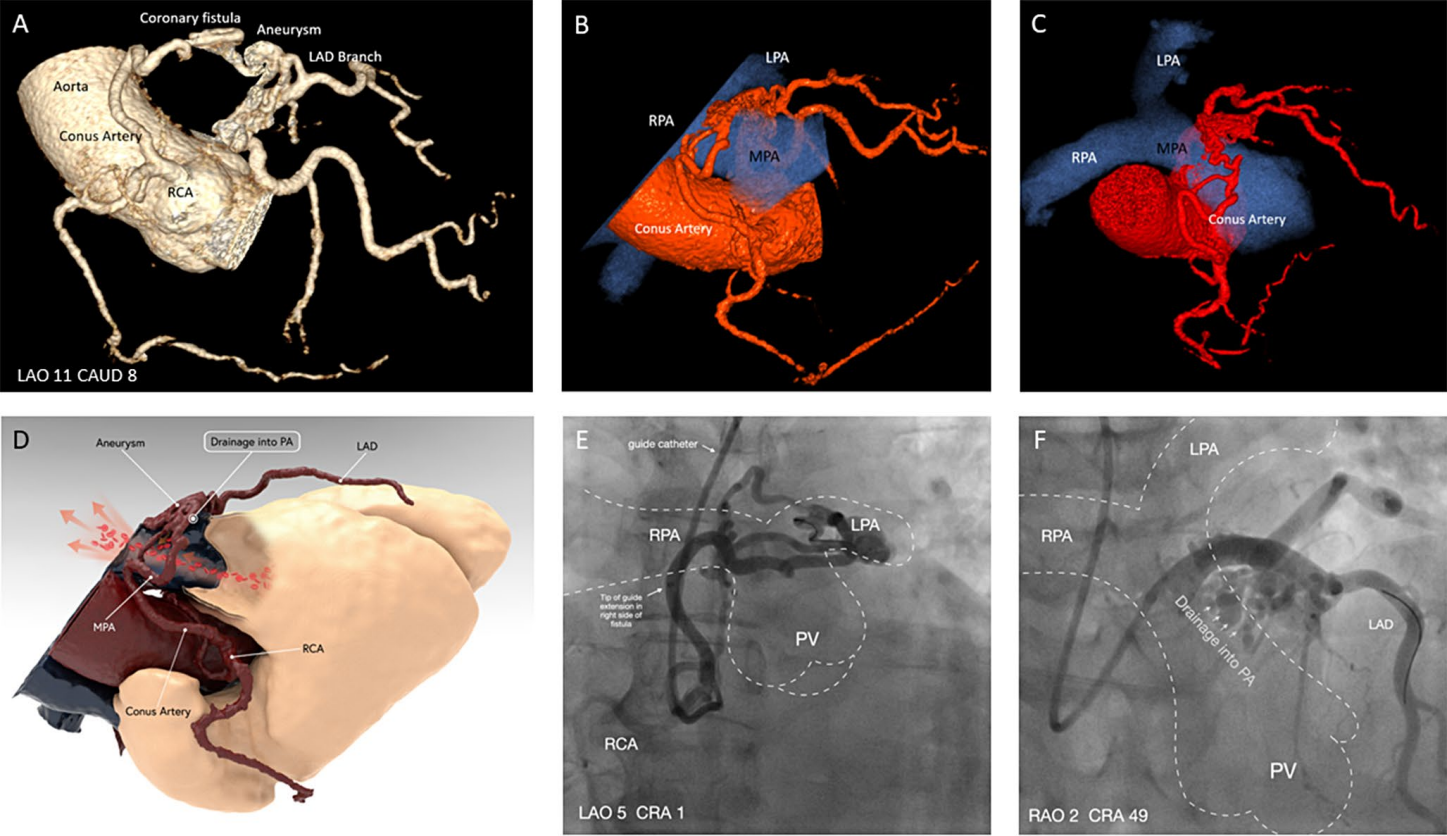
Panel (A) Coronary computed tomography angiography 3D volume rendering of the coronary-to-coronary fistula forming a complete circle around the main pulmonary artery (MPA not displayed). (B, C) Computed tomography 3D model showing the anatomy of the coronary-to-coronary fistula and its relationship with the pulmonary arteries. (D) Computed tomography 3D volume rendering of the fistula in relation to MPA. (E,  F) Invasive coronary angiography in different angulations with sketch of course of the pulmonary arteries and site of drainage of the coronary fistula into the main pulmonary artery
